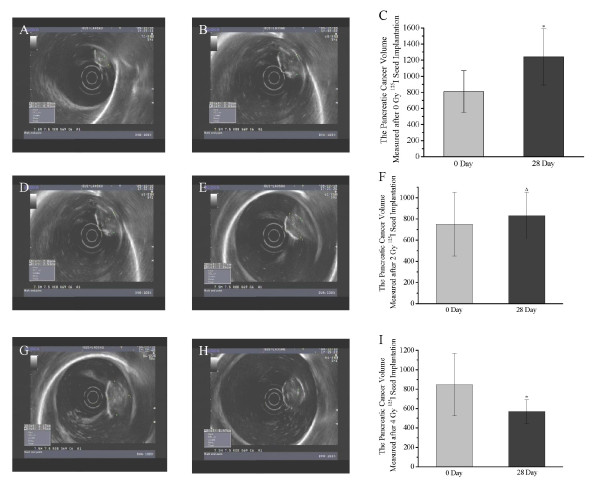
Tumor volume 0 and 28 d after ^125^I seed implantation. The upper, middle, and lower panels show representative ultrasound images from 0 Gy (upper), 2 Gy (middle), and 4 Gy (lower) groups 0 and 28 d post ^125^I seed implantation. P *< 0.05 compared with 0 d post-implantation; ^Δ^*P *> 0.05 compared with 0 d post-implantation.
